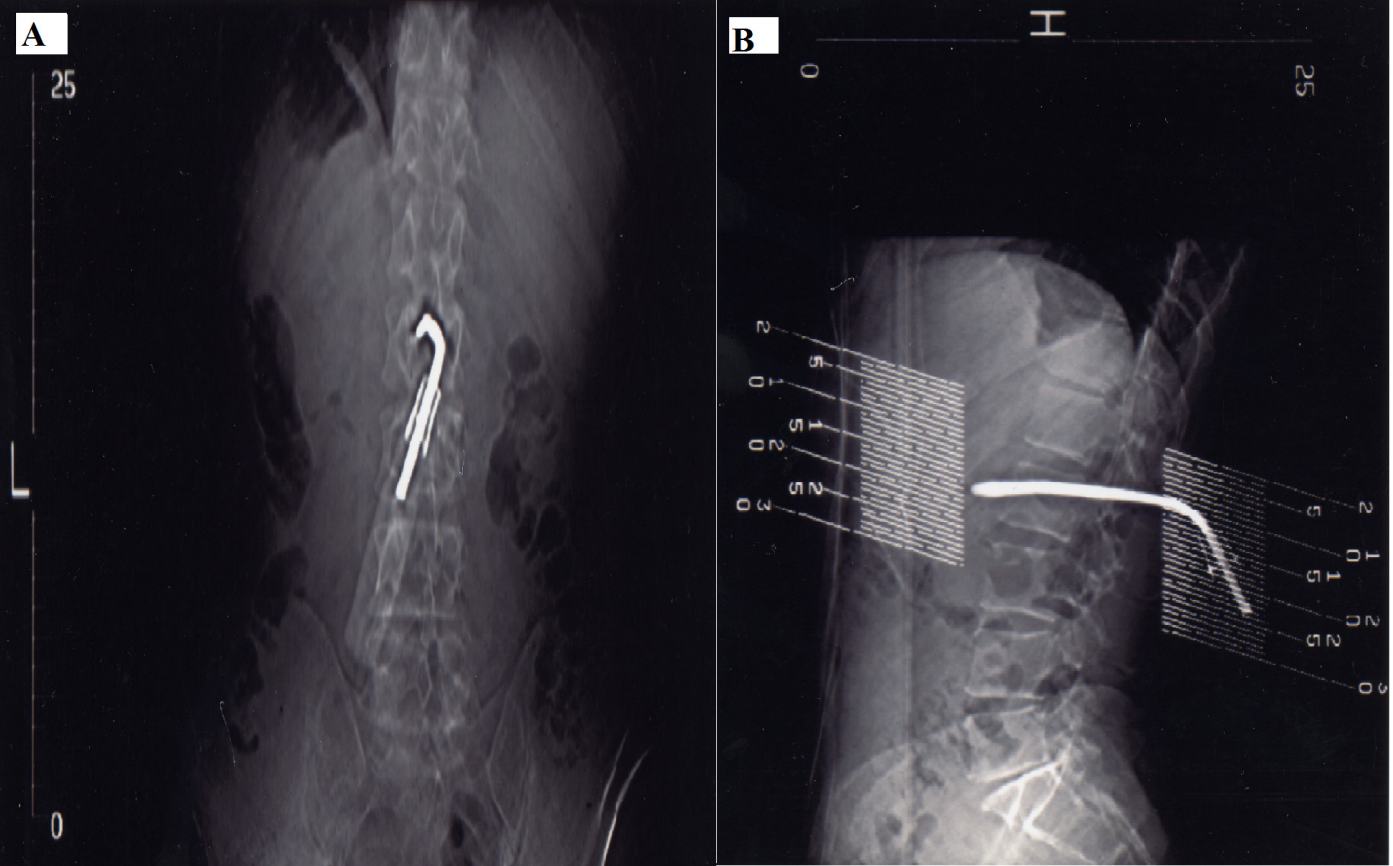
(A) AP and (B) Lateral CT scan of abdomen demonstrating position of screwdriver.
